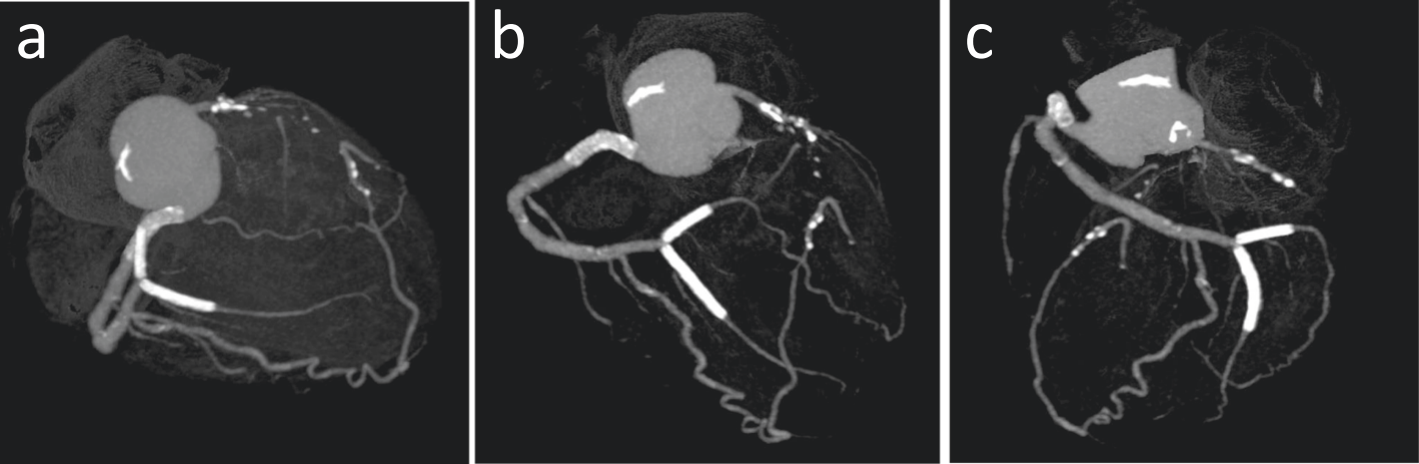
Coronary computed-tomography angiography (CTA). CTA was performed after elective percutaneous coronary intervention (PCI) to the distal branches of the right coronary artery (RCA) (#4:posterior lateral branch and atrio ventricular branch). a Right anterior oblique (RAO)-cranial view, b Anteroposteriol-cranial view, c Left anterior oblique (LAO)-cranial view. The right posterior descending artery communicated directly with the left anterior descending artery (LAD) through the apex
